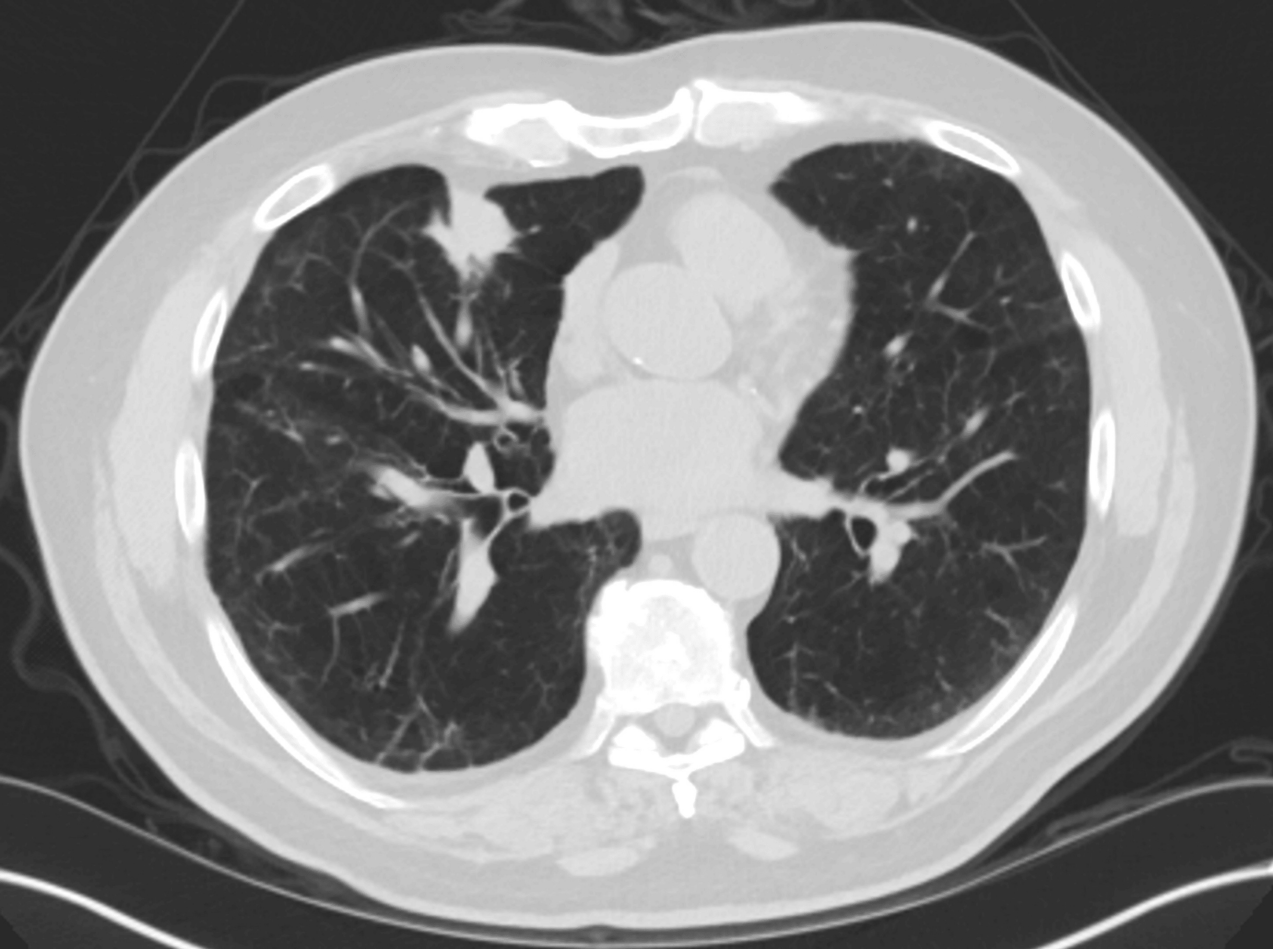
Chest CT January 2024: After Two Cycles of Treatment Abbreviations: CT, computed tomography
